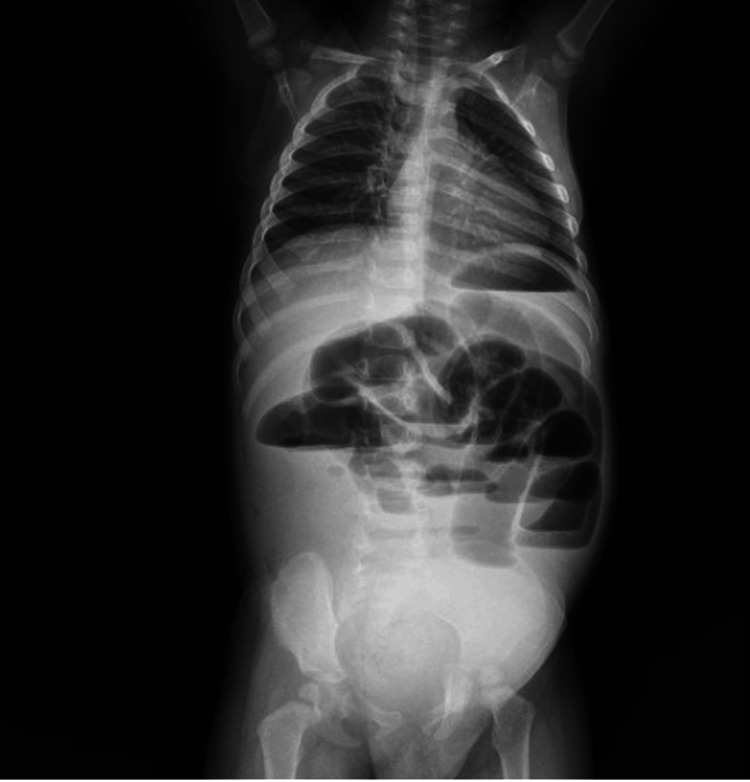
Abdominal X-ray anteroposterior projection in the standing position The abdominal X-ray reveals intestinal loop dilatation and gas-filled loops.
